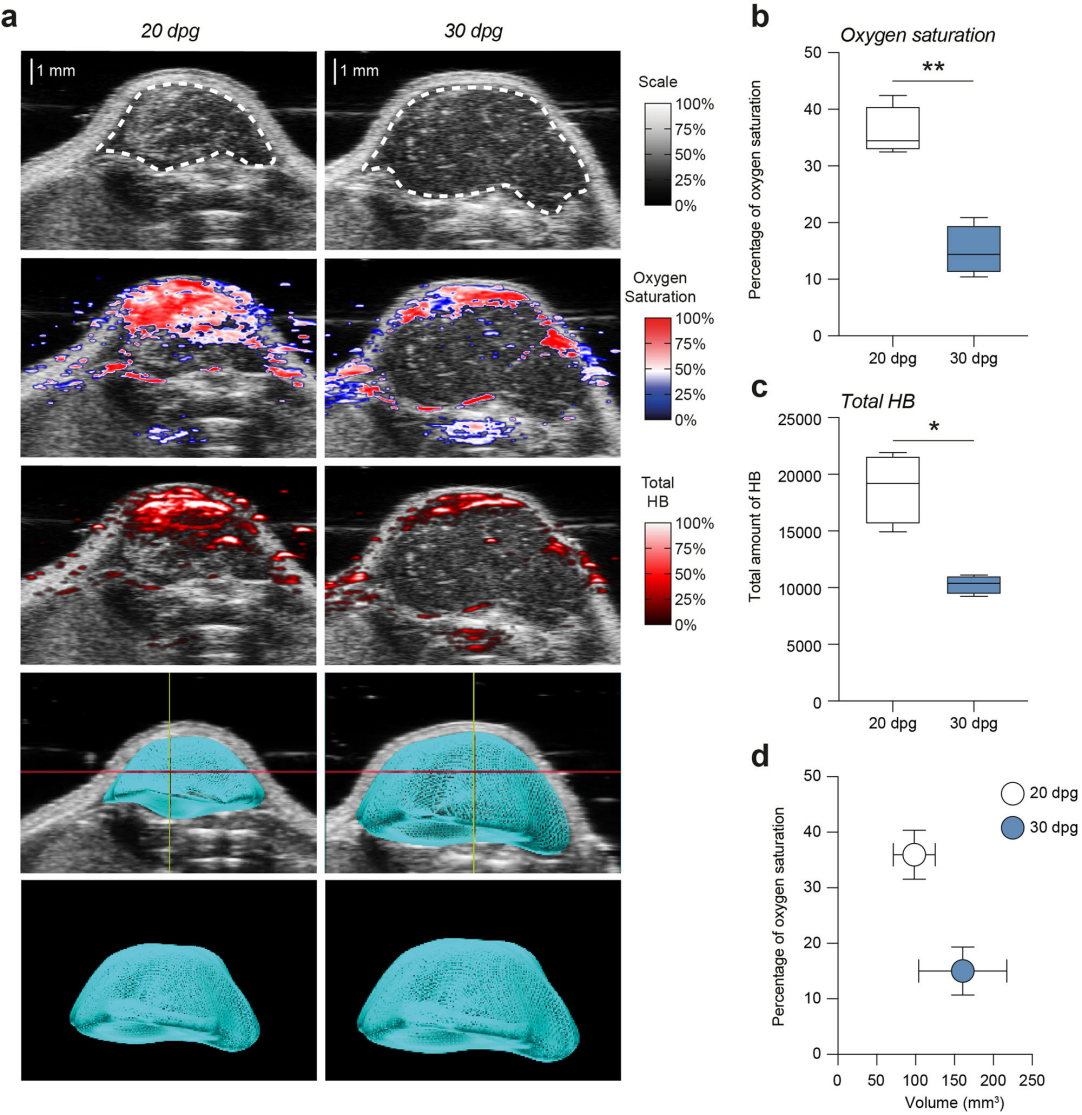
Tumor oxygenation and hemoglobin are reduced overtime and inversely correlate with tumor growth. a Ultrasound-photoacoustic assisted imaging of heterotopic GBM showing representative 2D frames tumor mass, oxygen saturation, total hemoglobin and 3D reconstruction of tumor mass at 20 and 30 dpg. b–c Quantification of oxygen saturation and total hemoglobin levels within the tumor mass at 20 (white boxes and white circle) and 30 (blue boxes and blue circle) dpg. d Biplot of the percentage of oxygen saturation and tumor volume expressed in mm^3^. p*-value < 0.05 and *p*-value<0.01. HB hemoglobin, dpg: days post-graft.
